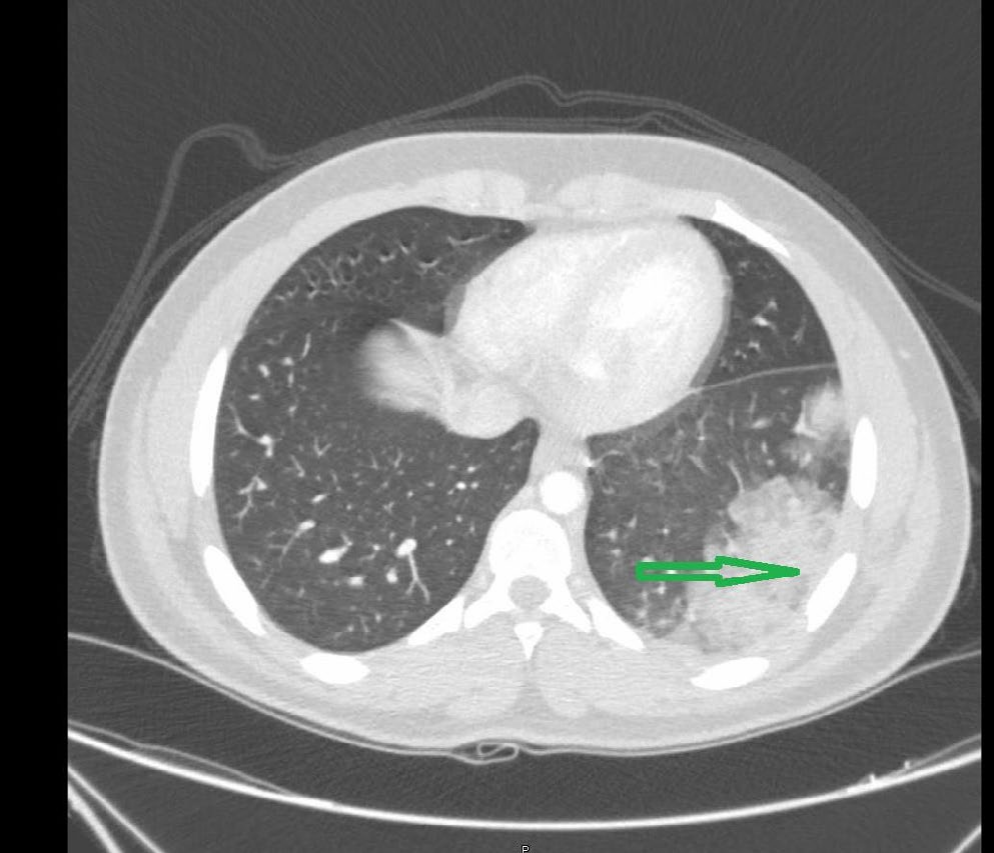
CT chest showed ‘left lower lobe opacities that may be secondary to lung contusion/alveolar hemorrhage’
